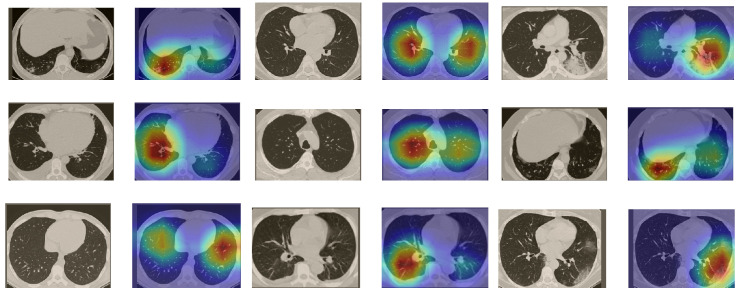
Grad-CAM visualizations for examples of CT images from the SARS-CoV-2 dataset. Our InceptionV3 model correctly classified them as COVID-19 and localized the most relevant regions used for its decision. The first, third, and fifth columns show CT images with COVID-19 findings, whereas the second, fourth, and sixth columns represent their corresponding localization maps generated by Grad-CAM.
